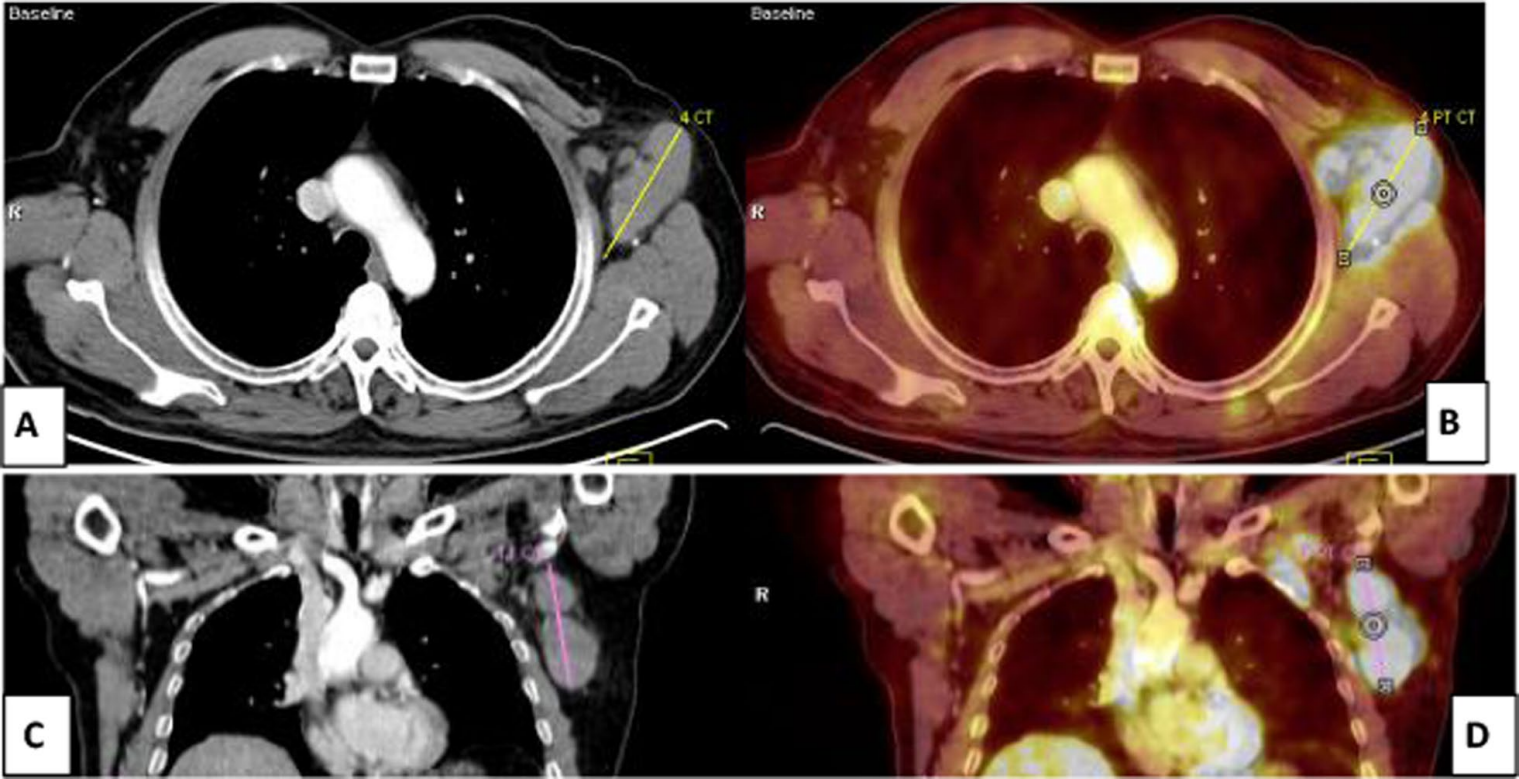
A 45-year-old male patient with NHL, treated with anti-cd20 antibody rituximab 25 months prior to vaccination. The most recent PET/CT showed enlarged amalgamated left axillary LNs. Further clinical data revealed that he got his 2nd dose of Pfizer-BioNTech vaccine 9 days before the PET-CT study. So, these findings were ascribed to recent vaccination. A Axial and C coronal CECT images revealed enlarged amalgamated left axillary LNs about 8 cm. B Axial and D coronal PET/CT images revealed FDG-avid left axillary LNs of 17 SUVmax
